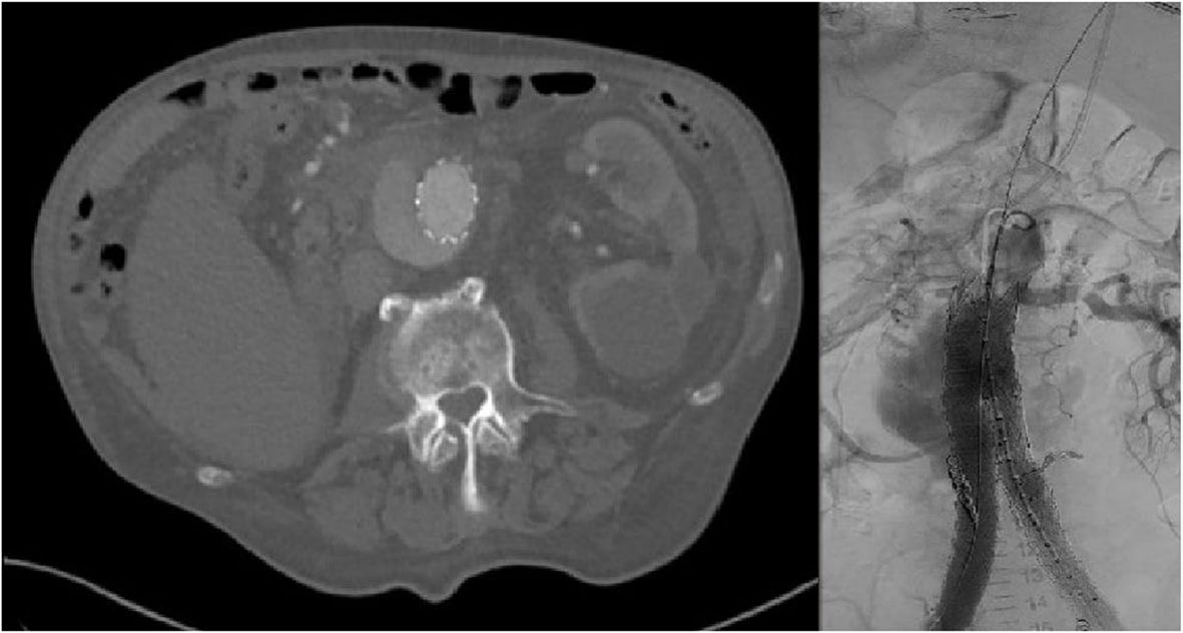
Type IA endoleak presenting 7 years after primary therapy (CTA (a), DSA (b))
